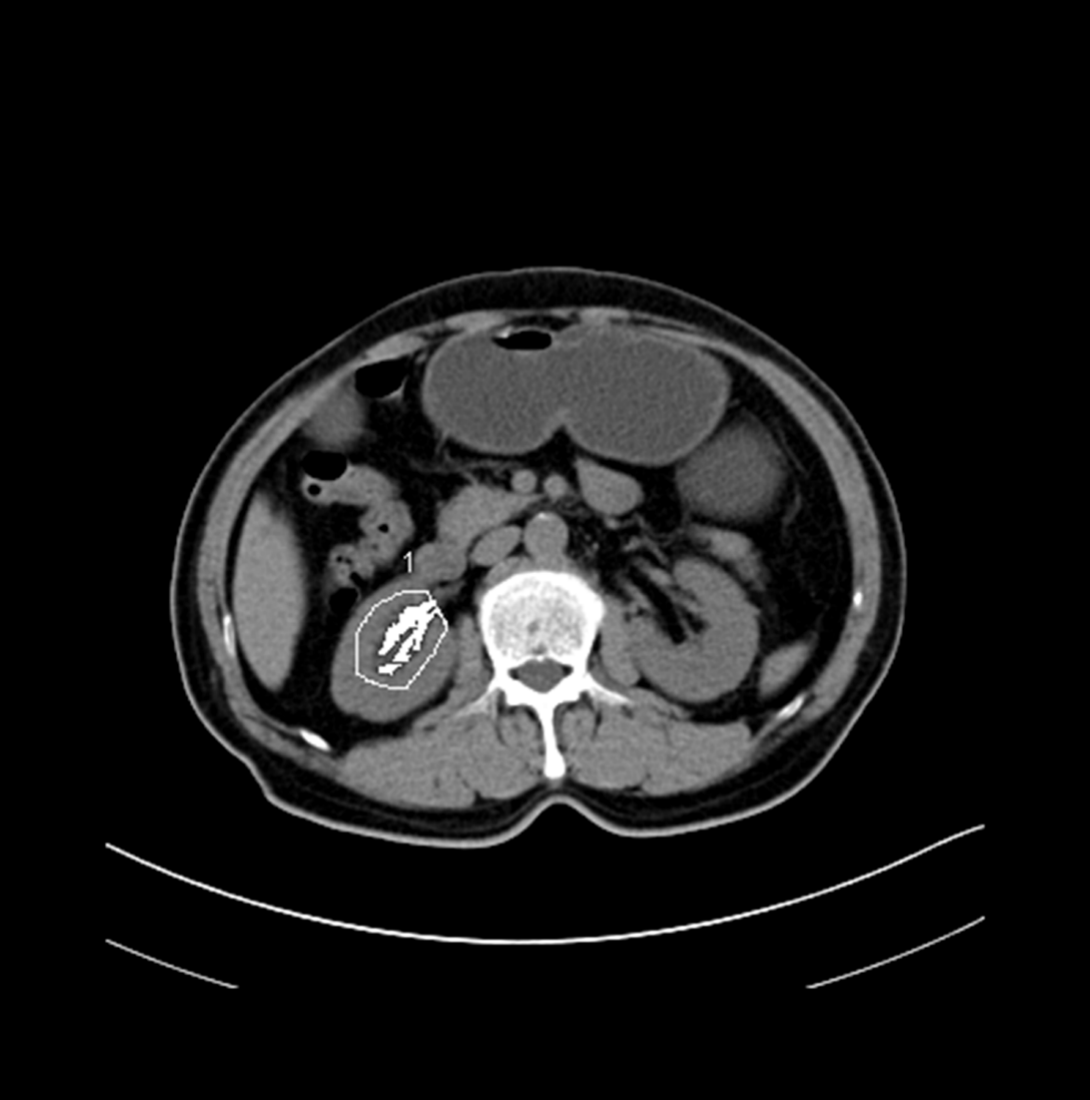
Representative axial CT image showing renal sinus fat area
